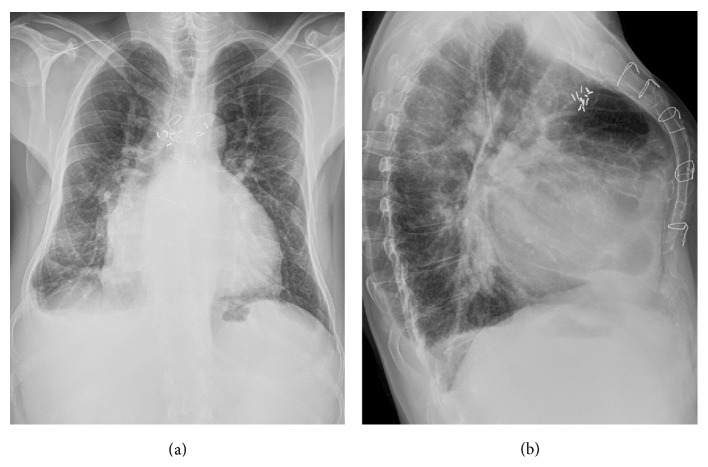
Two projection chest X-rays with the anteroposterior view on the left and the lateral view on the right, showing signs of pulmonary congestion and a right basal pleural effusion.
